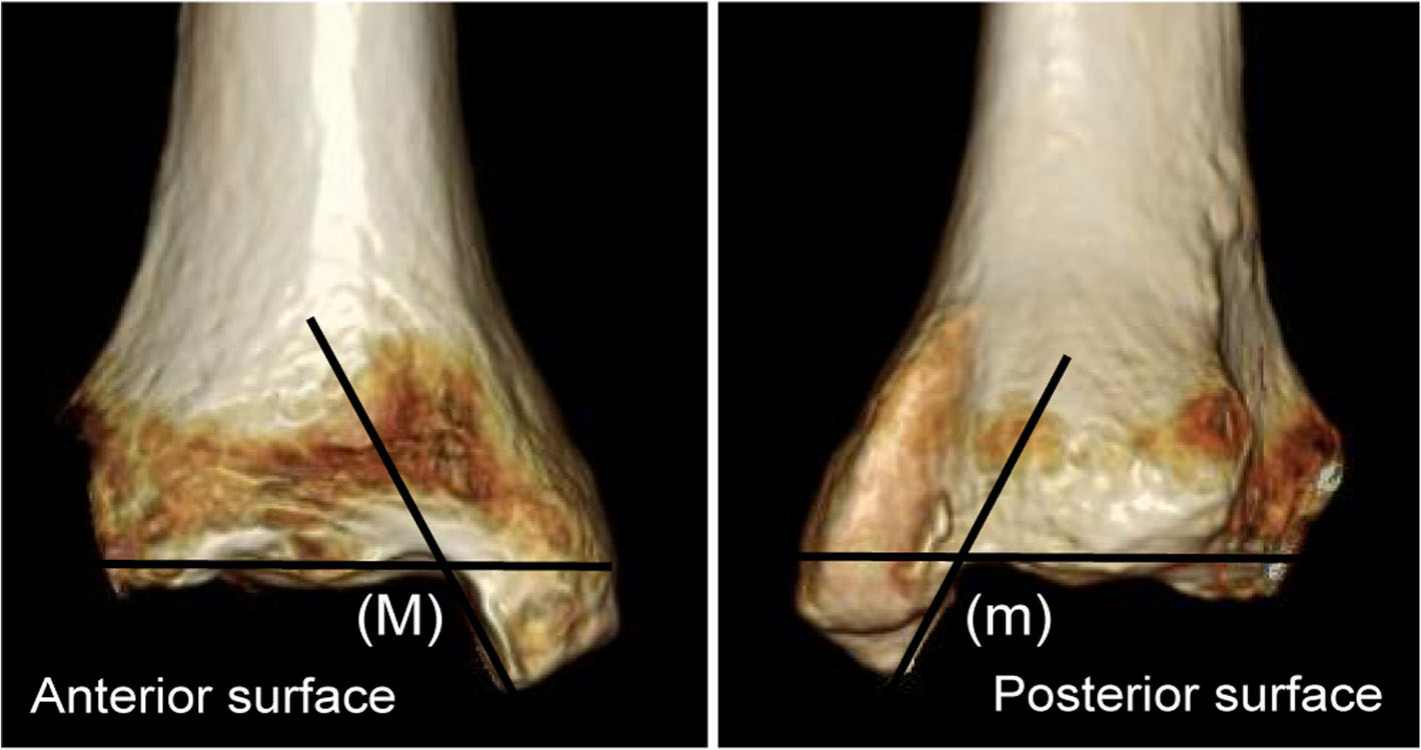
Anterior and posterior three-dimensional computed tomography images of the distal articular surface . M: Intersection point of the tangent of the distal anterior articular surfaces and the malleolar articular surface on the anterior sides of the tibia, respectively. m: Intersection point of the tangent of the distal posterior articular surfaces and the malleolar articular surface on the posterior sides of the tibia, respectively
